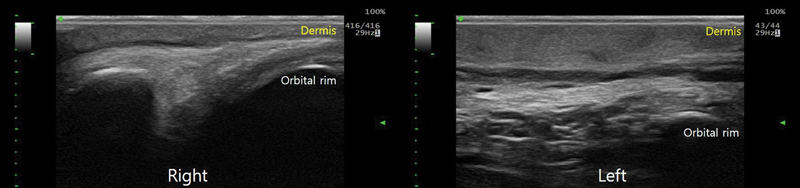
Ultrasonographic findings of a patient with left upper eyelid lymphedema showing thickened dermis and a coarse subcutaneous layer above the orbital rim.
